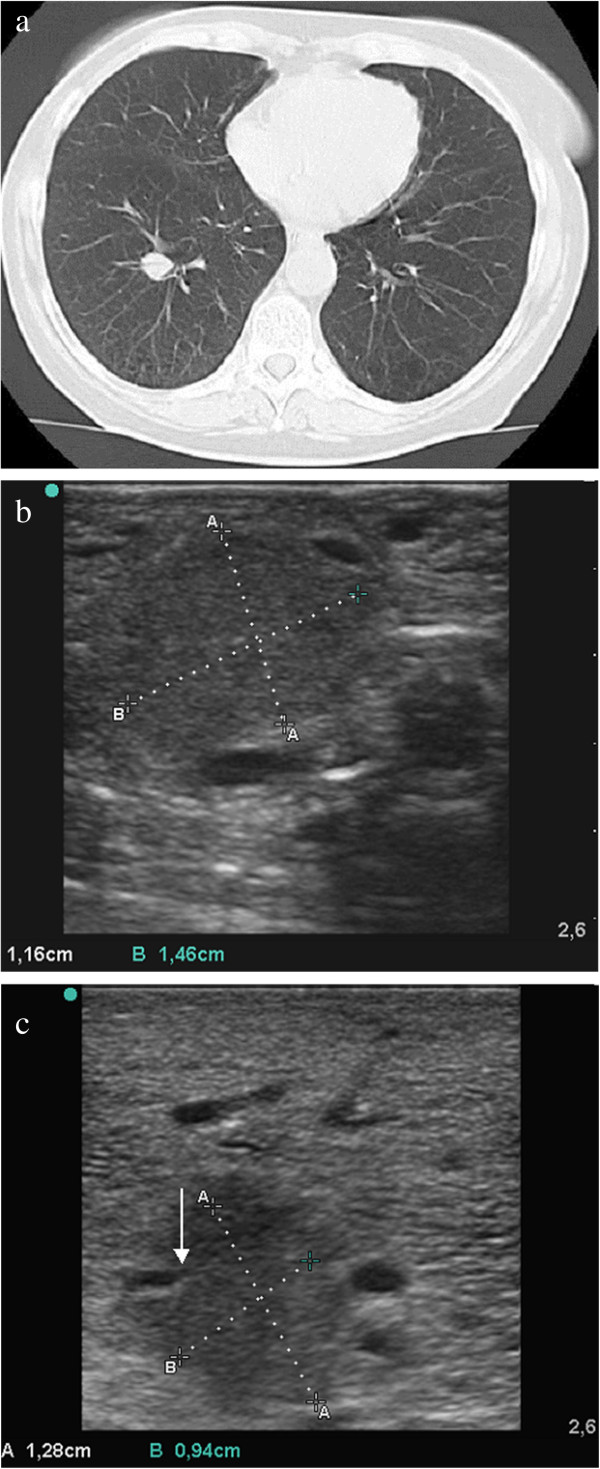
Adenocarcinoma in the central right inferior lobe of an excised human lung. (a) Computed tomography scan shows a central, peribrochial tumour. (b) Sonography under atelectasis: the tumour appears homogenous and is poorly demarcated (isoechogenic) from lung tissue. (c) Sonography after flooding: homogenous tumour tissue is hypoechoic, irregularly configured with fringe-like processes, and is well-demarcated from the normal lung parenchyma. Bronchial destruction is evident (arrow).
